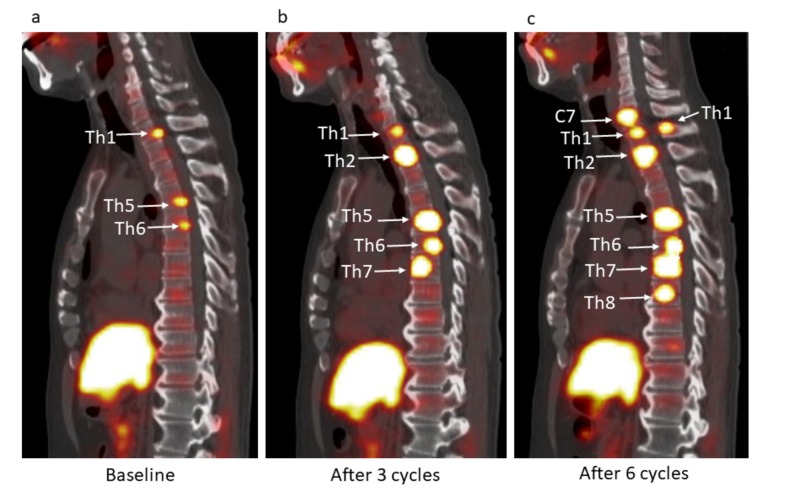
Selected sagittal 11C-choline PET/CT plane at (a) baseline, after (b) three and (c) six cycles. (a) Three hot spots were observed in Th1 vertebra (SUVmax, 4.9), Th5 vertebra (SUVmax, 4.7), and Th6 vertebra (SUVmax, 4.3) on pretreatment ^11^C-choline PET/CT (arrows), reflecting bone metastases. (b) Five hot spots were observed in Th1 vertebra (SUVmax, 4.9), Th2 vertebra (SUVmax, 15.0), Th5 vertebra (SUVmax, 18.1), Th6 vertebra (SUVmax, 12.8), and Th7 vertebra (SUVmax, 13.2) on ^11^C-choline PET/CT after three cycles (arrows), reflecting the progression of bone metastases. (c) Eight hot spots were observed in C7 vertebra (SUVmax, 11.1), Th1 vertebra (SUVmax, 5.7) Th1 spinous process (SUVmax, 6.2), Th2 vertebra (SUVmax, 14.4), Th5 vertebra (SUVmax, 16.2), Th6 vertebra (SUVmax, 12.0), Th7 vertebra (SUVmax, 12.6), and Th8 vertebra (SUVmax, 12.2) on ^11^C-choline PET/CT after six cycles (arrows), reflecting further progression of bone metastases.
